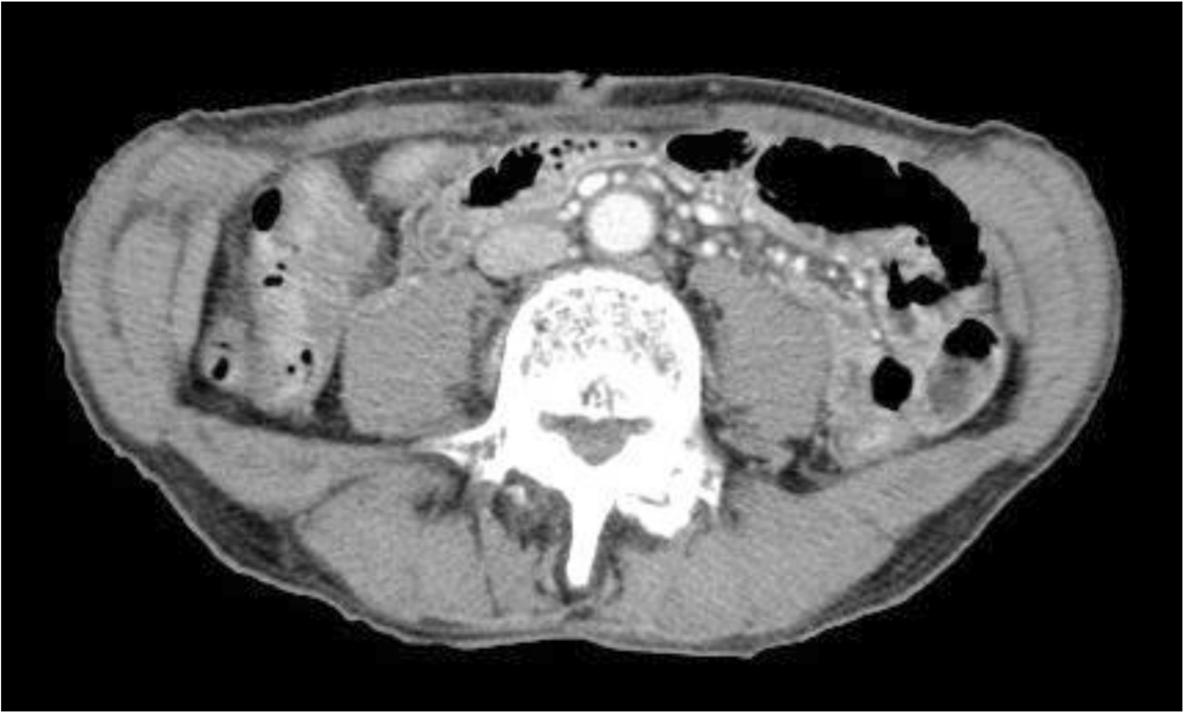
CT performed 10 months after surgery shows no evidence of the recurrence of ascites
